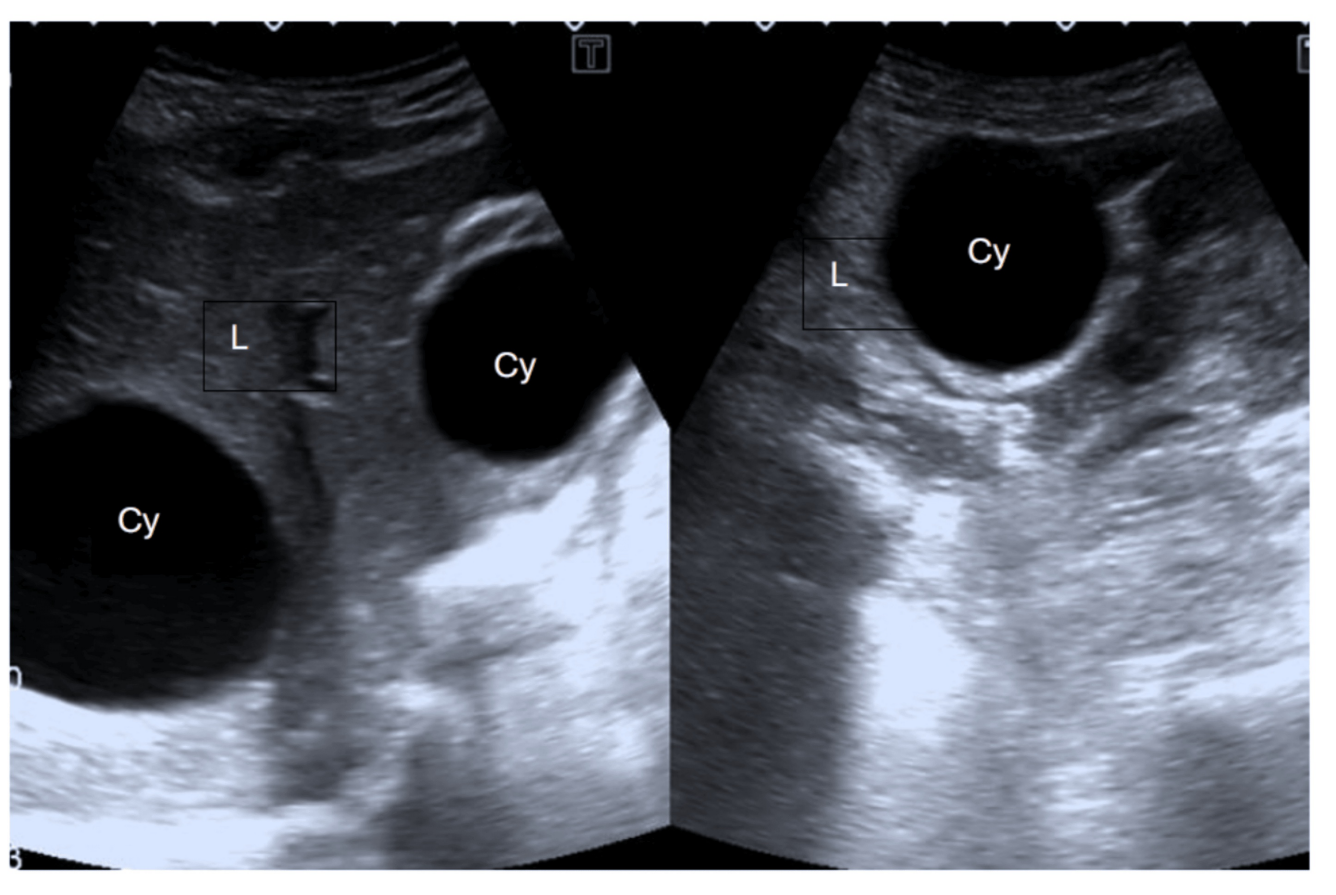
Ultrasound of the abdomen showing hydatid cysts (Cy) persisted in the liver (L) after treatment with albendazole.
